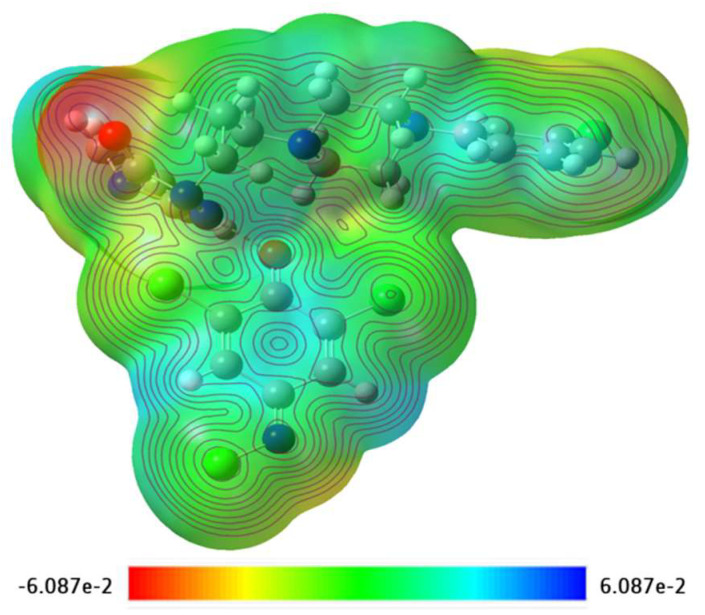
MEP surface map of the CT complex [(TZD)(DCQ)] with respective color scales.
